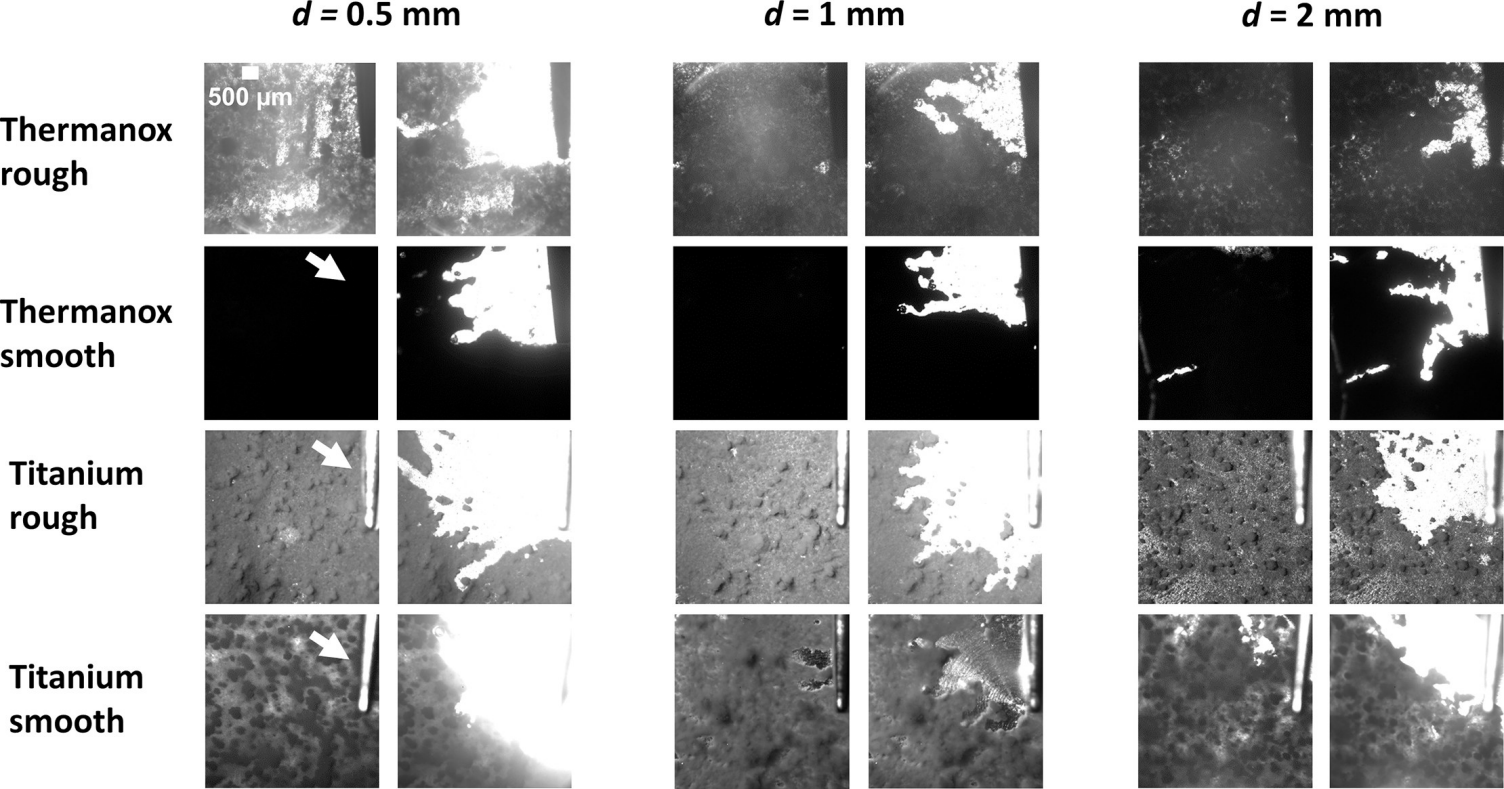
High speed image stills before and after cavitation cleaning for 2 s for various biofilm surfaces and standoff distances of the ultrasonic scaler tip. The white arrow shows the position of the scaler tip in the images. The white area is the cleaned surface and the grey/black area is the biofilm still attached to the surface. See S1 Video for high speed videos of all surfaces at all standoff distances.
